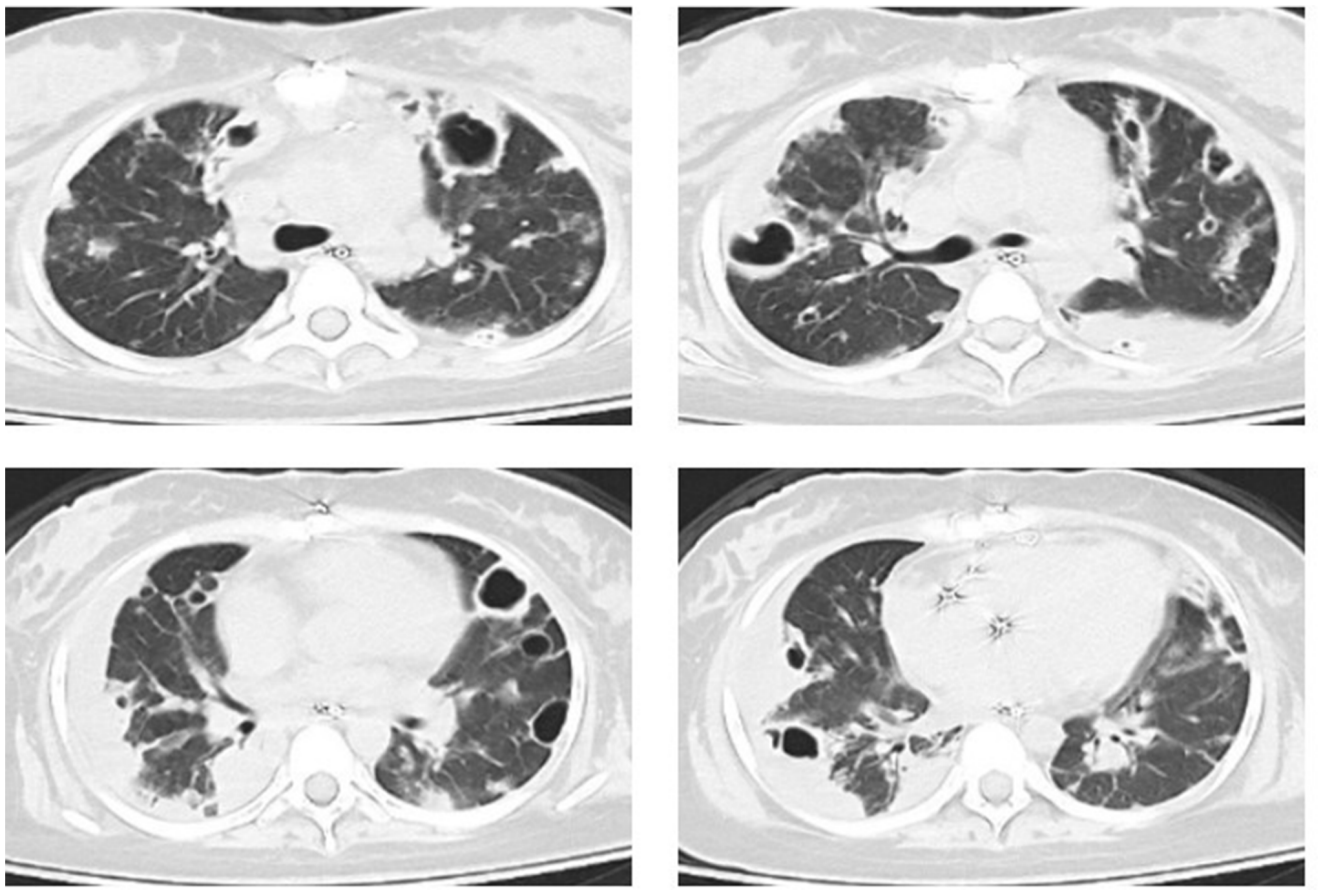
CT scan showed multiple cavitary infiltrates in both lungs on admission.
